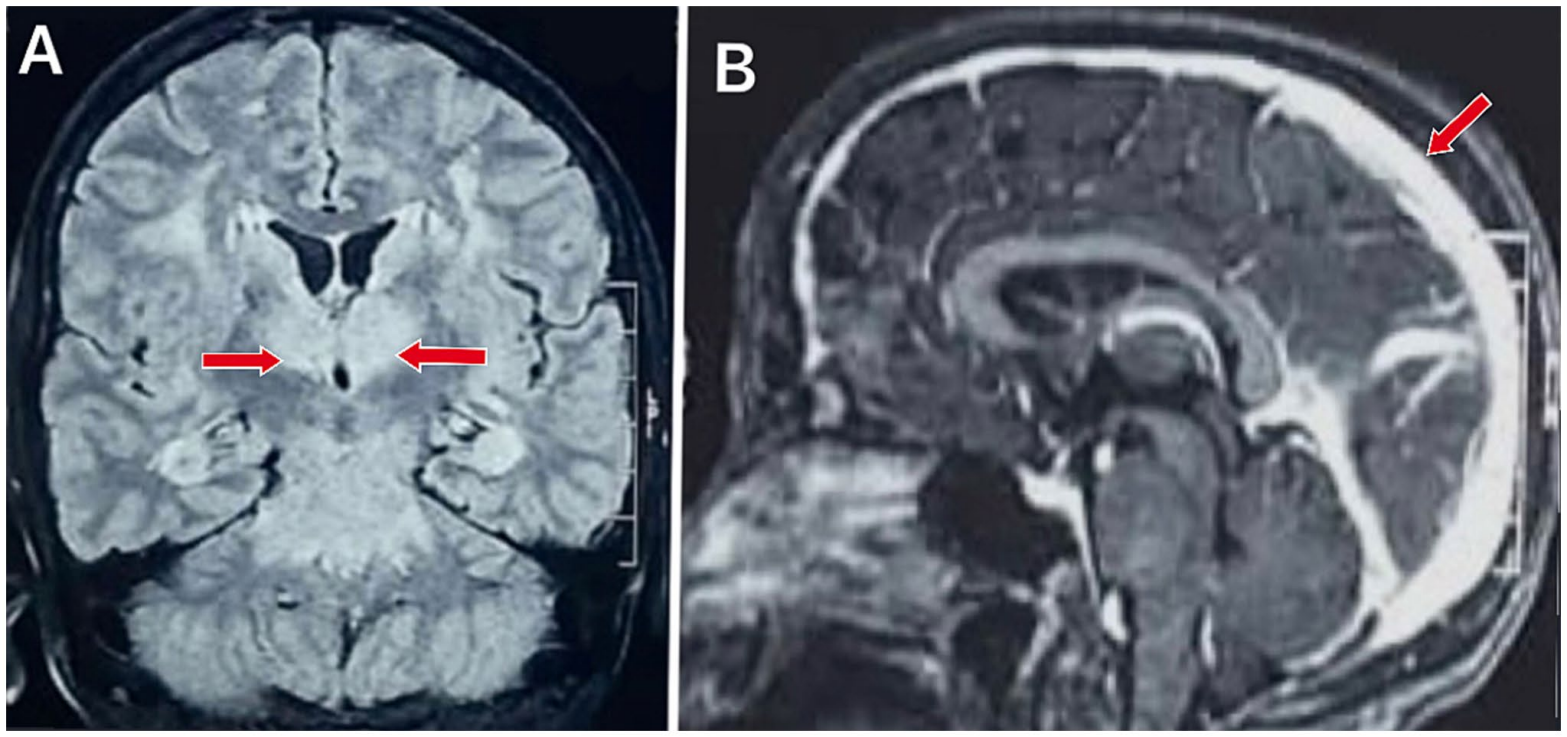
Coronal and sagittal MRI images of the brain show pathological findings: (A) Coronal Fluid-Attenuated Inversion Recovery (FLAIR) sequence demonstrates bilateral symmetrical hyperintense lesions in the thalamus (red arrows). (B) Sagittal post-contrast T1-weighted image shows contrast enhancement in the meninges (red arrow), suggesting meningeal involvement.
